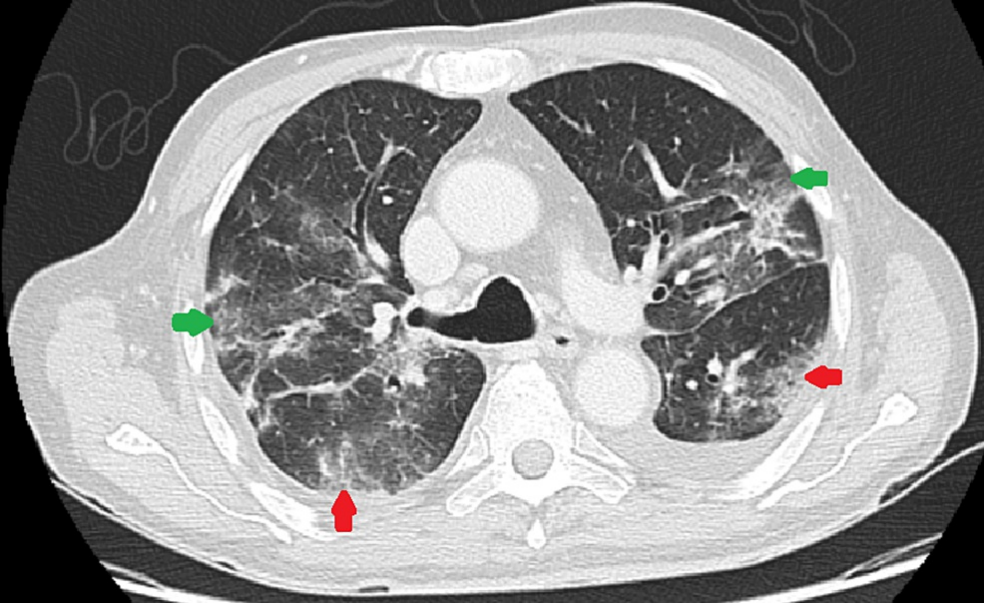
Thoracic CT scan performed in April 2021 Green arrows: fibrosis and bronchiectatic evolution of the previously affected pulmonary segments; red arrows: new ground-glass infiltrates in previously spared areas.
